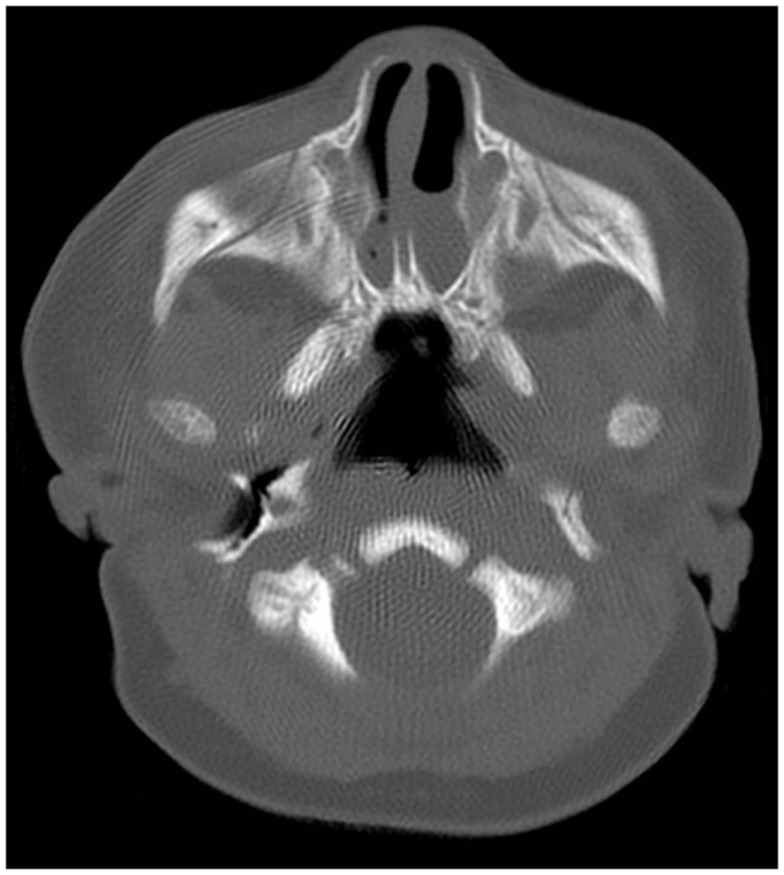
Axial CT scan of the nasal cavity in bone window at the level of choana.
